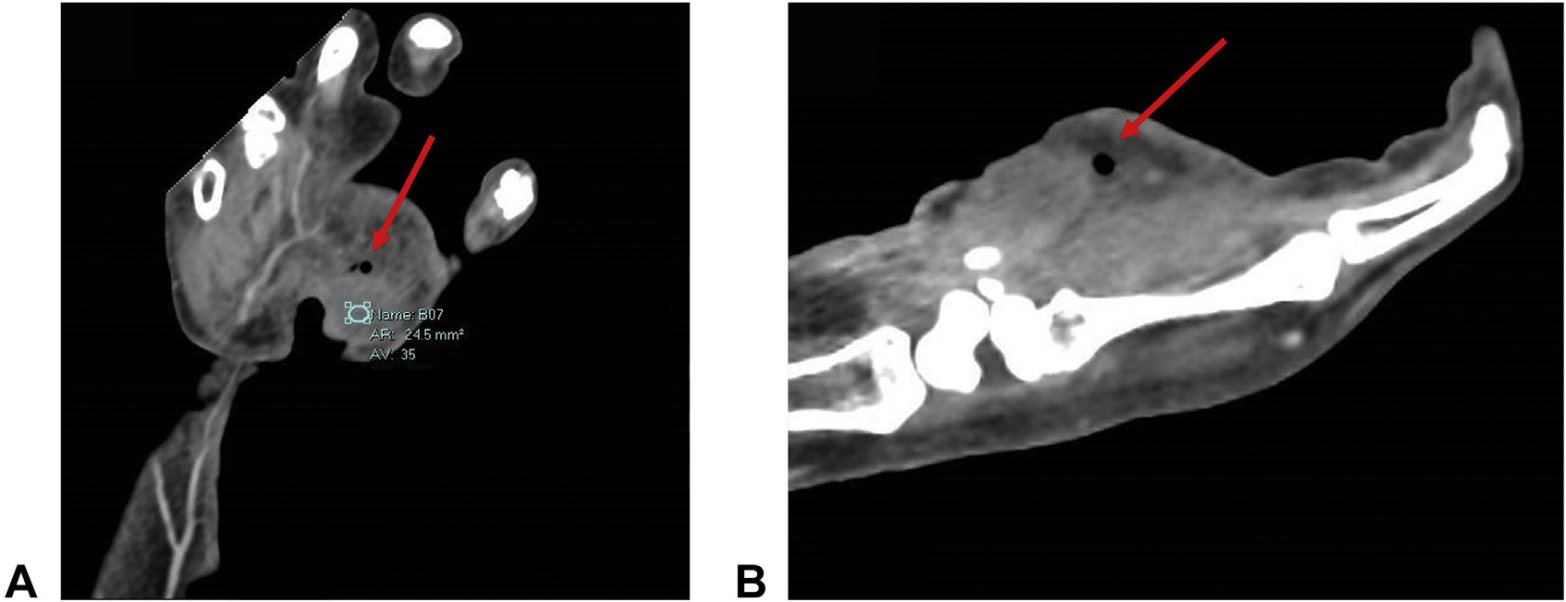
X-ray revealing phlegmon and gas formation in the distal thenar eminence (red arrows). A Coronal image. B Sagittal imagef.
